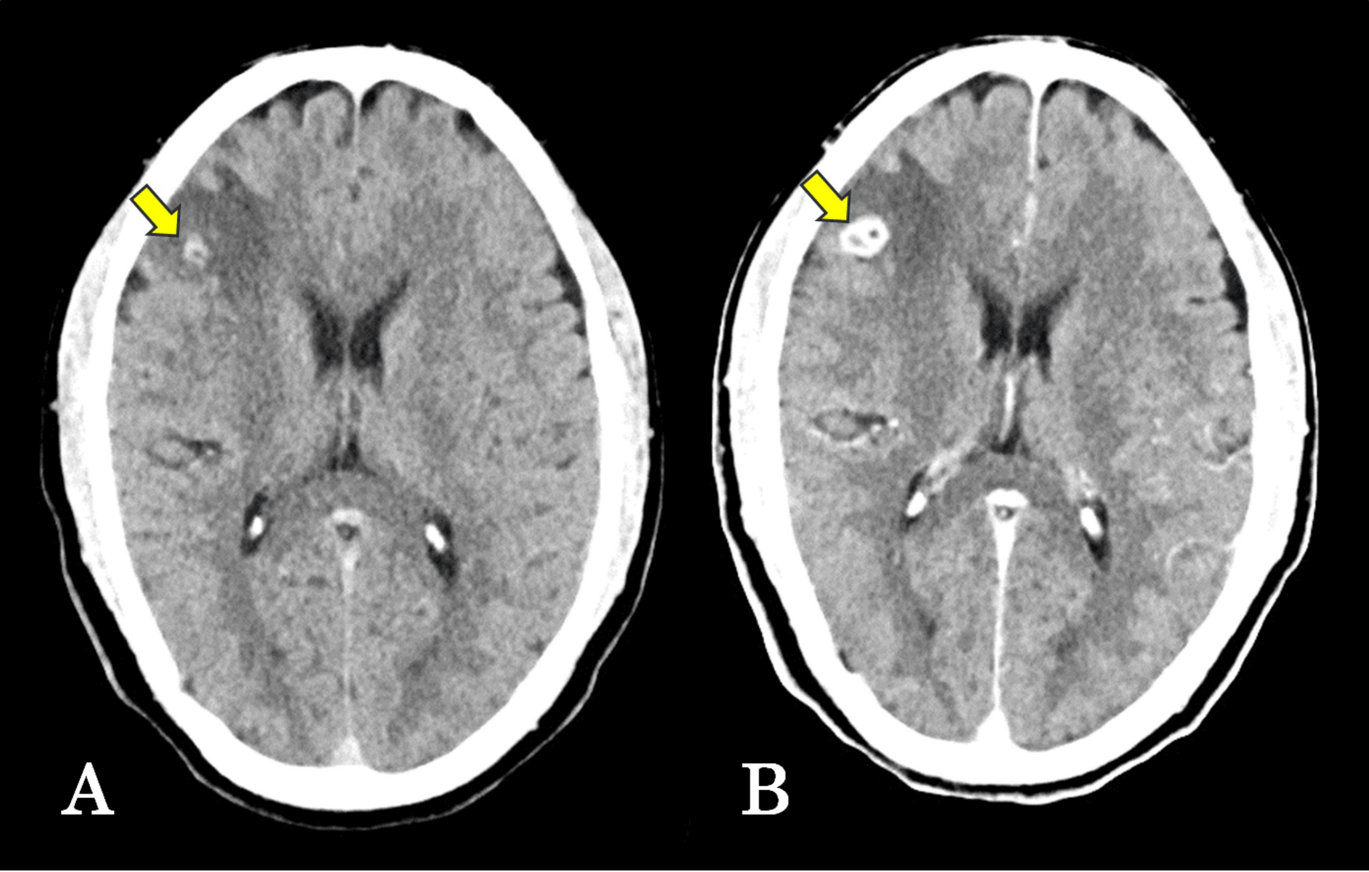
Computed tomography (CT) findings of the intracranial lesion (A) Non-contrast head CT showing a calcified mass lesion in the right frontal lobe. (B) Contrast-enhanced CT demonstrating ring enhancement of the lesion. The arrows indicate the lesion.
